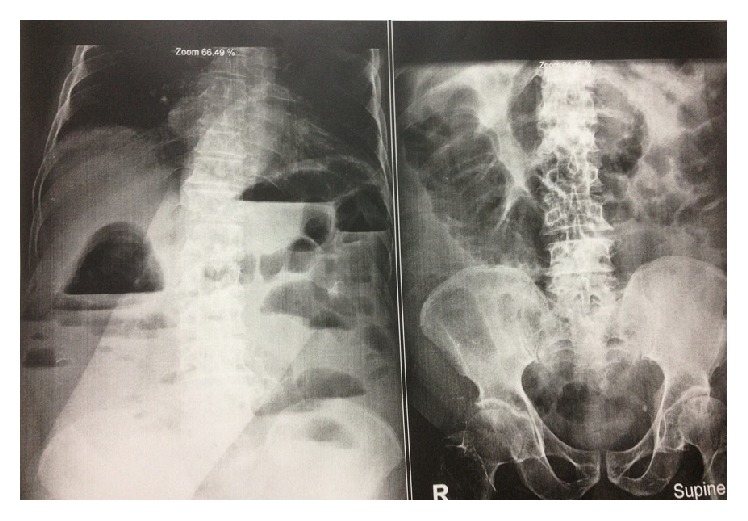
Abdominal X-ray (supine and upright) just before surgery.
